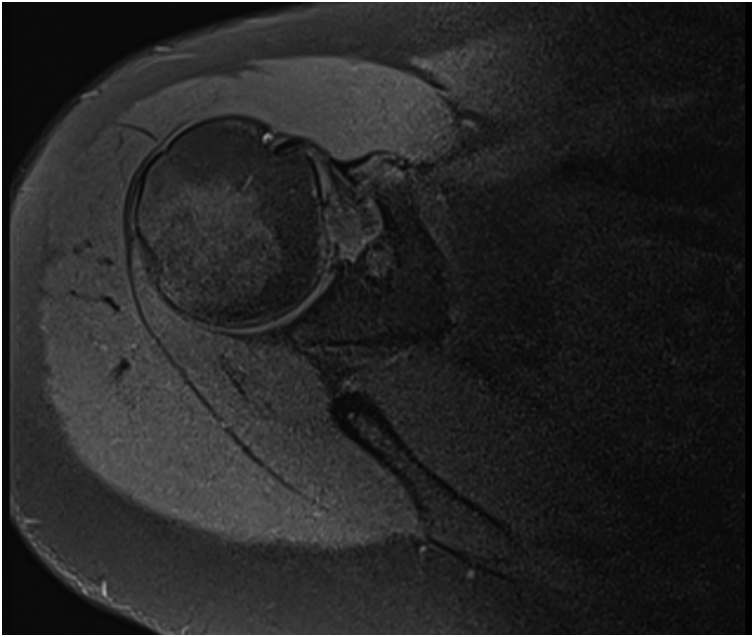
Non-contrast axial MRI of the right shoulder.
